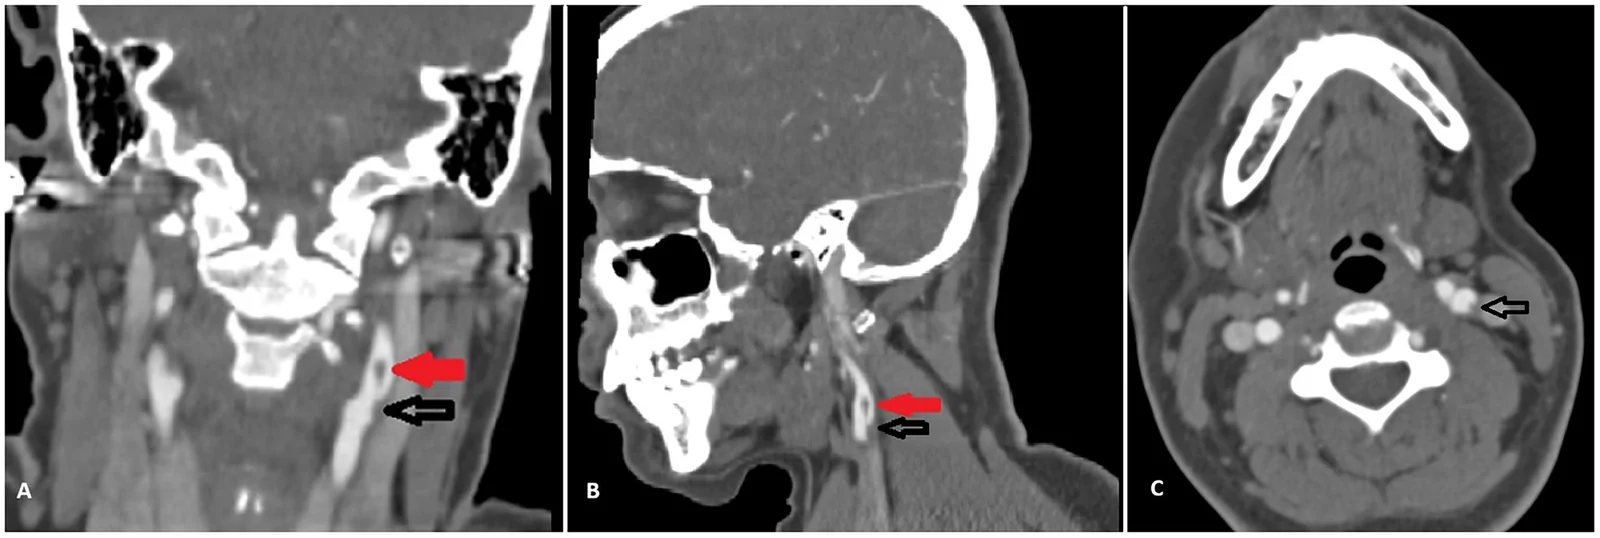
CT angiography of the supra-aortic vessels showing a shelf-like intraluminal filling defect arising from the posterior wall of the left internal carotid artery, just distal to the carotid bifurcation, consistent with a carotid web (black arrow), associated with an intraluminal thrombus (red arrow). Coronal (A), sagittal (B), and axial (C) reconstructions.
